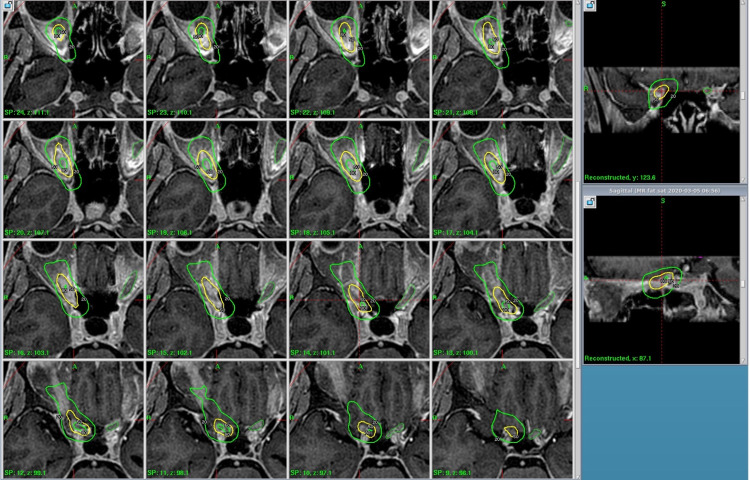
GKRS treatment plan. Axial T1 contrast-enhanced MRI with GKRS plan showing the 80% isodose, 50% isodose, and 20% isodose lines with the plan along and adjacent to the optic nerve. The prescription dose remained at 15 Gy to the 50% isodose line. GKRS: Gamma Knife radiosurgery; MRI: magnetic resonance imaging
